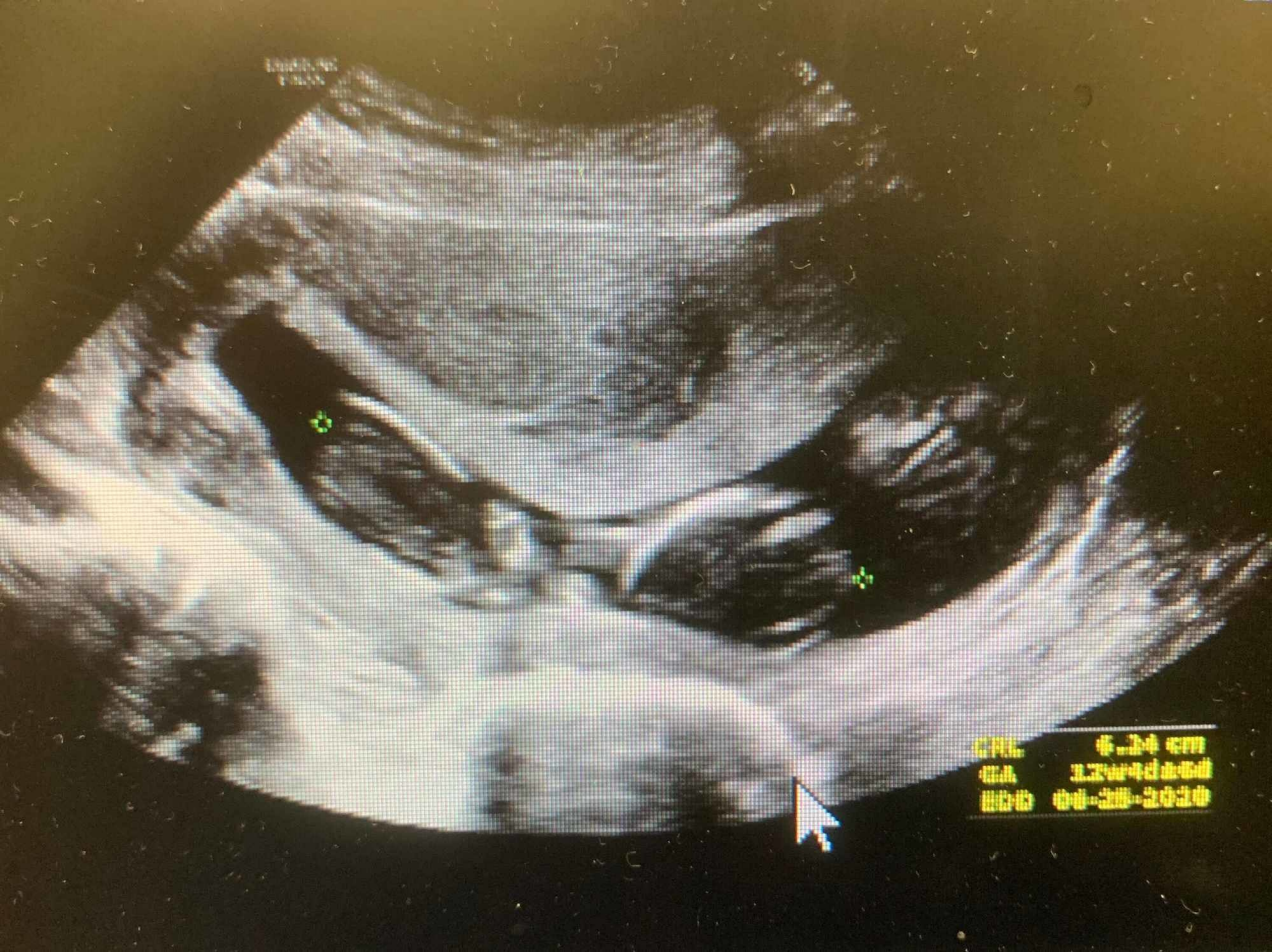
Ultrasound pelvis found an abnormal appearing fetus without a cardiac activity, estimated GA of 12 weeks, and four days GA, gestational age.
